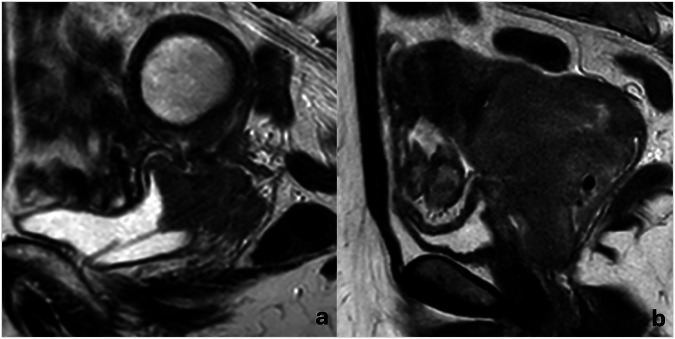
Two cases of MRI-defined bladder wall invasion on sagittal FSE T2-weighted images. a A complete loss of neoplasm-bladder cleavage plan, a massive bladder wall thickening with intermediate signal intensity, as well as endoluminal tumor growth, is appreciable; a DJ catheter was placed because of hydronephrosis. b An incomplete loss of neoplasm-bladder cleavage plan and a discrete bladder wall thickening with intermediate signal intensity is appreciable, but no endoluminal tumor growth can be observed. The first case was positive at cystoscopy, whereas the second one was negative. Both patients underwent local tumor recurrence (13 and 19 months after chemoradiation, respectively), and both died (25 and 36 months after initial diagnosis, respectively)
